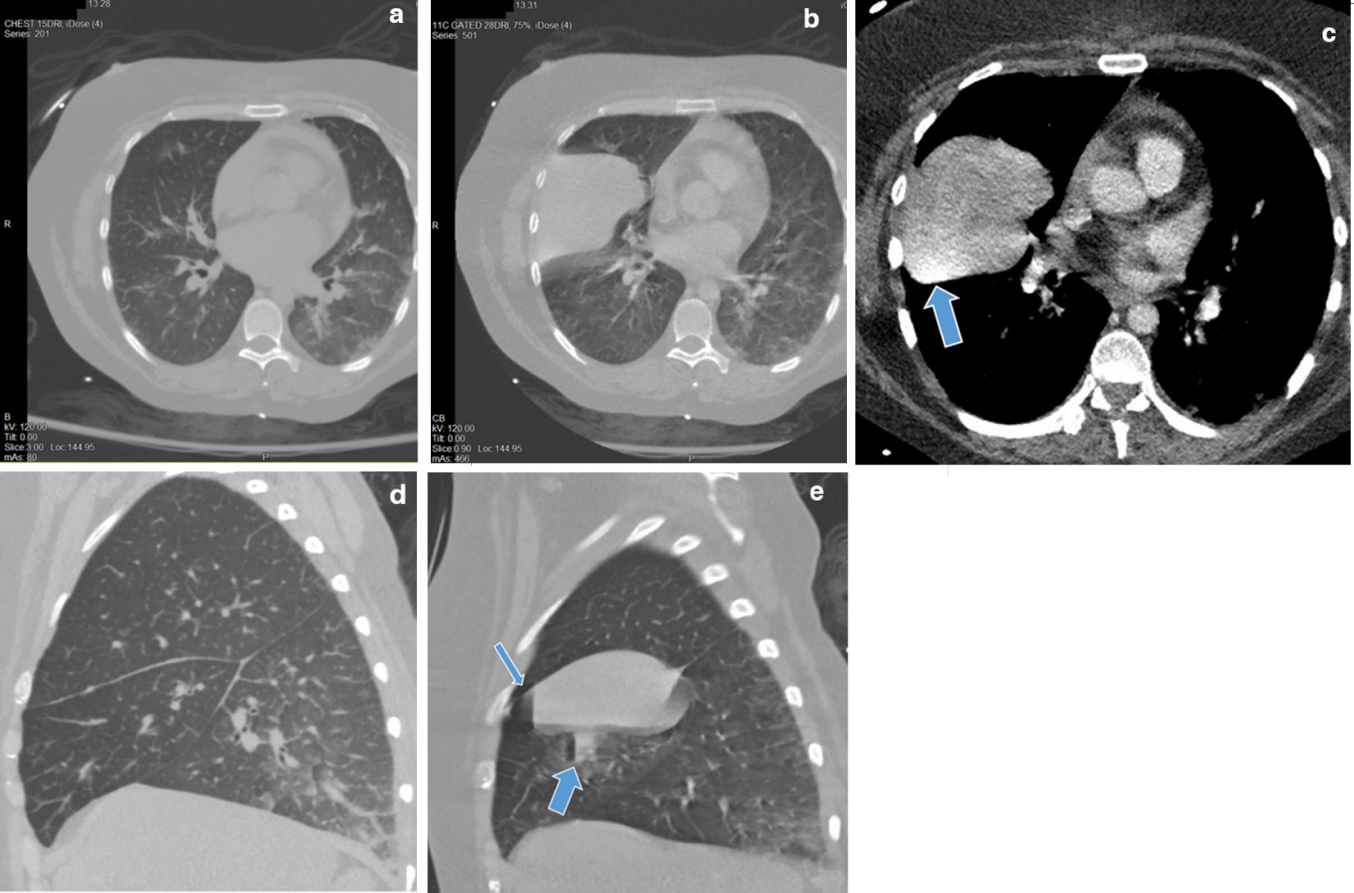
(A-E). CT angiography images obtained on date of injury. (A) Axial CT pre-contrast image at the level of the middle lobe showing no evidence of hemorrhage in this region. (B) CT angiography image obtained within 3 min of the pre-contrast imaging showing a new, large, dense collection filling the pleural space subjacent to the minor fissure. This was not present 3 min earlier on pre-contrast imaging (3A). Note the timestamp in the right upper corners of the images. (C) CT angiography image set to soft-tissue windowing better demonstrates a region of increased density at the most dependent portion of the collection with attenuation similar to contrast (arrow), confirming active extravasation of recently given contrast media. (D, E) Pre-contrast and angiograhy sagittal reformats better demonstrating lack of hemorrhage or pneumatocele/air cyst formation prior to contrast injection, with sudden spontaneous parenchymal hemorrhage on angiography imaging. Note the air-fluid level within the right middle lobe parenchyma (wide arrow) suggesting traumatic air cyst formation with immediate hematoma development. A second air-fluid level is seen in the pleural space (thin arrow) consistent with subsequent hemopneumothorax formation.
